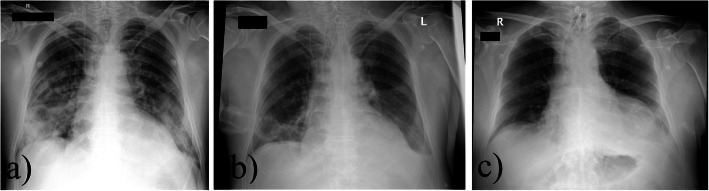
Anteroposterior chest X-rays of the patient on day 1 (a), 7 (b), 15 (c). a Day 1, confluent atelectasis on the day of admission. Central venous cannula and endotracheal tube in correct position. b Day 7, control examination. No signs of consolidation or atelectasis. c Day 15, control examination. No signs of consolidation or atelectasis. Tracheostomy tube in control position
